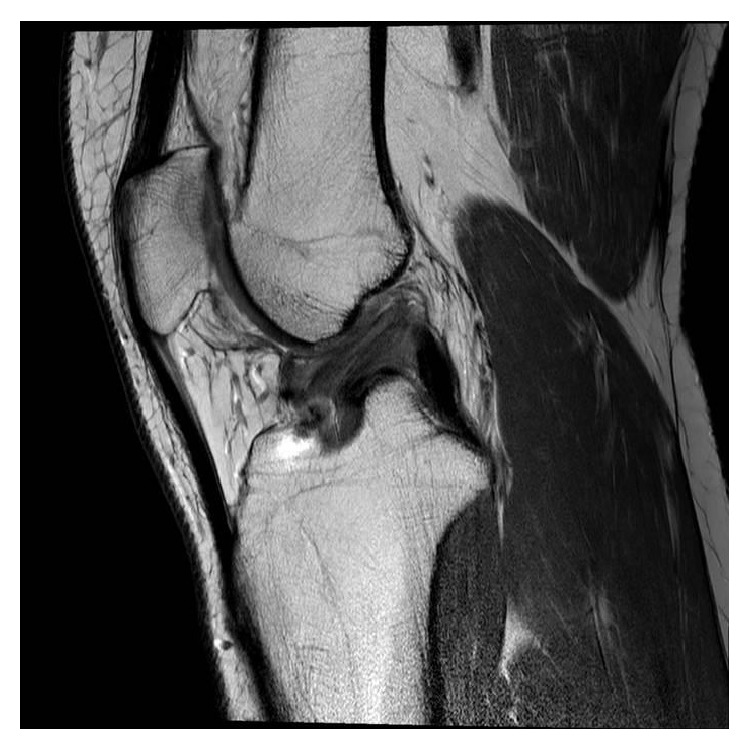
Postoperative sagittal MRI scan of the left knee at 16 months.
